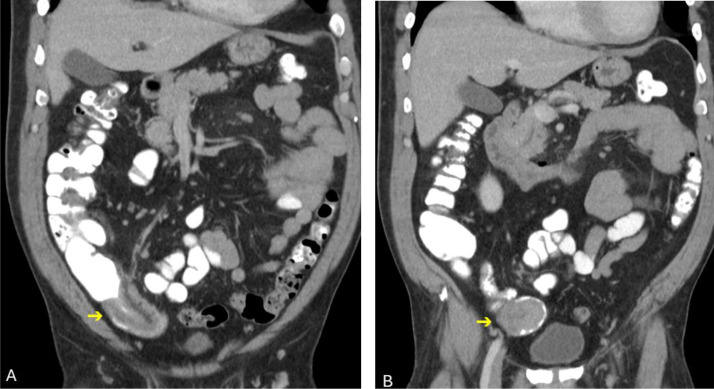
Coronal contrast-enhanced CT of the abdomen demonstrates ileo-ileal intussusception in the right lower quadrant (A). The intraluminal polypoid lead-point mass is clearly visualized (arrow, B), outlined by a circumferential rim of contrast*,* which highlights its intraluminal origin.
